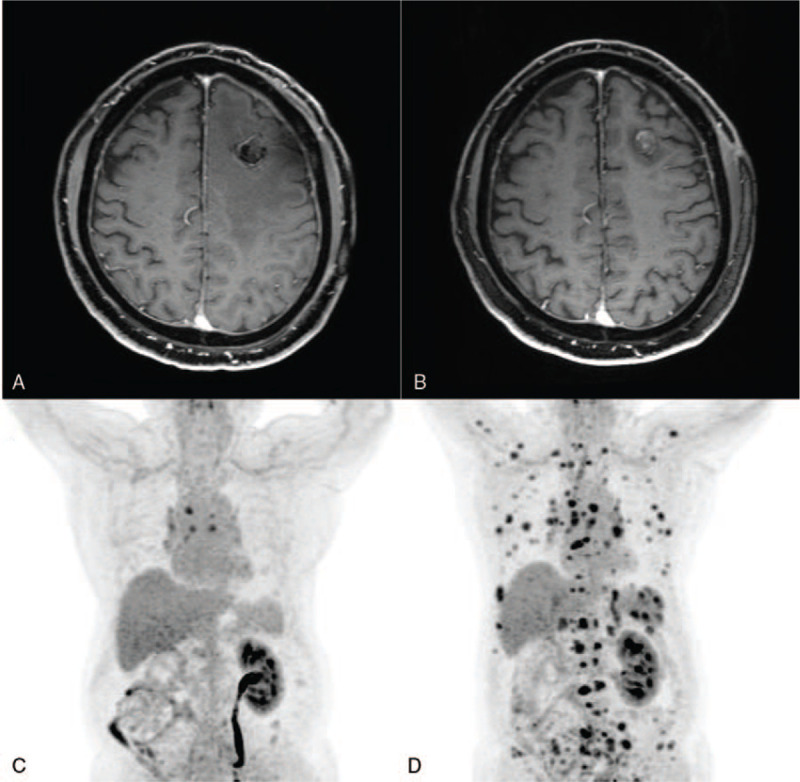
Series of magnetic resonance imaging (MRI) and whole-body positron emission tomography (PET) images. (A) Postoperative gadolinium-enhanced T1-weighted axial MRI performed a day after operation showing no evidence of remnant tumor. (B) Follow-up MRI at 4 mo after craniotomy. Gadolinium-enhanced T1-weighted axial MRI images showed no evidence of recurrence and decrease of peritumoral edema. (C) PET images taken at the time of diagnosis of brain metastasis. (D) Six months after surgery, follow-up PET was performed during chemotherapy. Multiple metastases were identified in the whole body.
